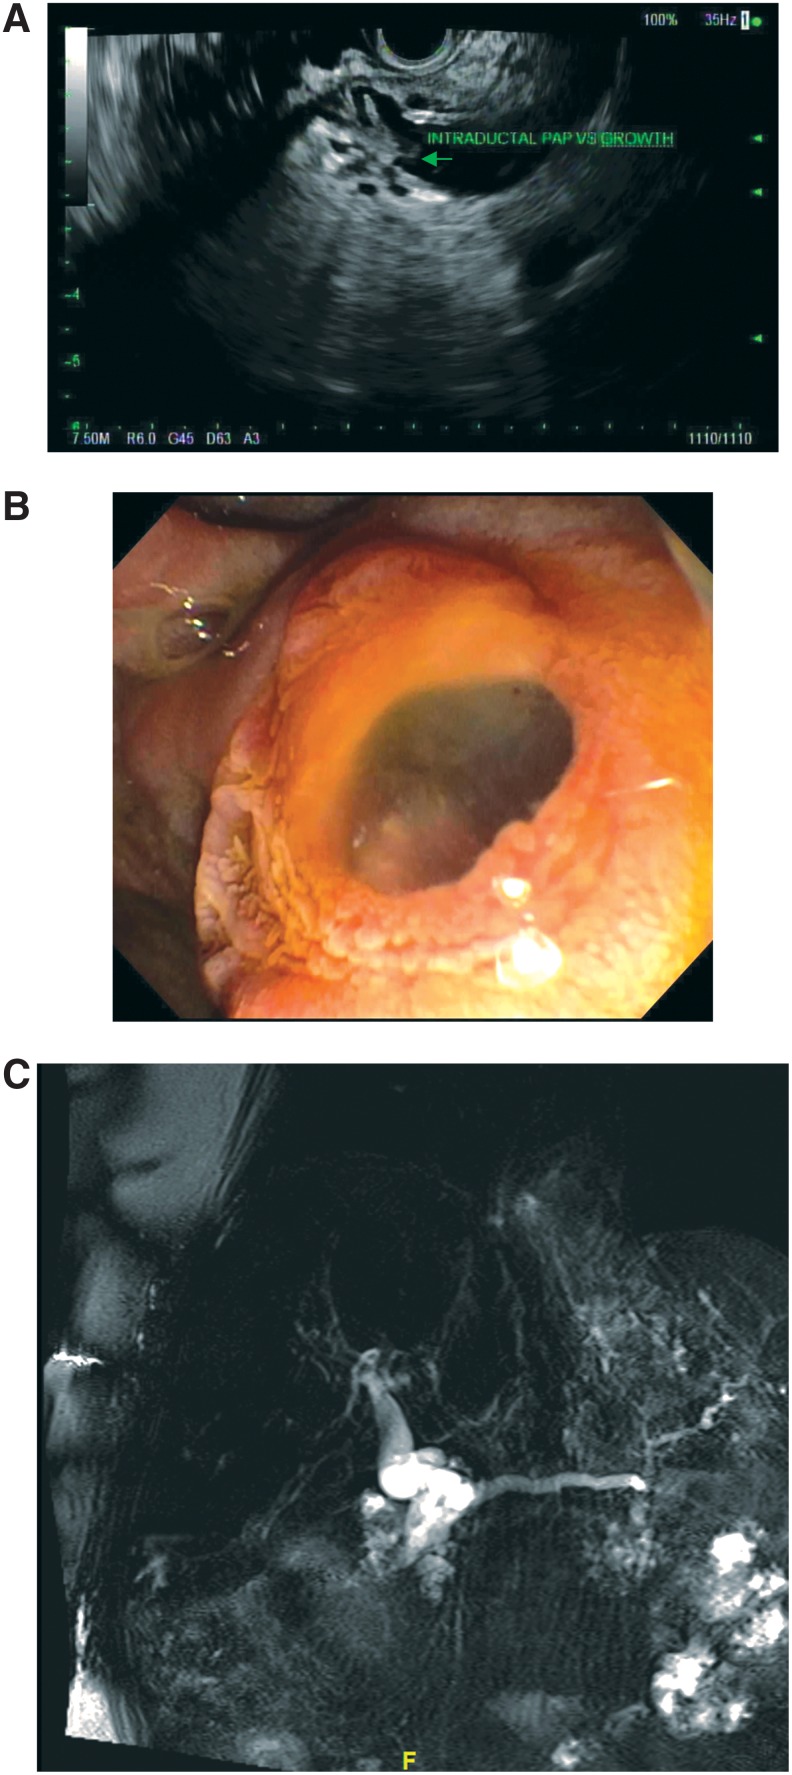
(A) Endoscopic ultrasound demonstrating a papillary growth in a dilated pancreatic duct in the head of the pancreas of the first patient. (B) Endoscopic image of the first patient's major duodenal papilla with mucin extrusion, suggestive of IPMN. (C) Magnetic resonance cholangiopancreatography demonstrating the second patient's diffuse pancreatic dilatation. IPMN, intraductal papillary mucinous neoplasm.
